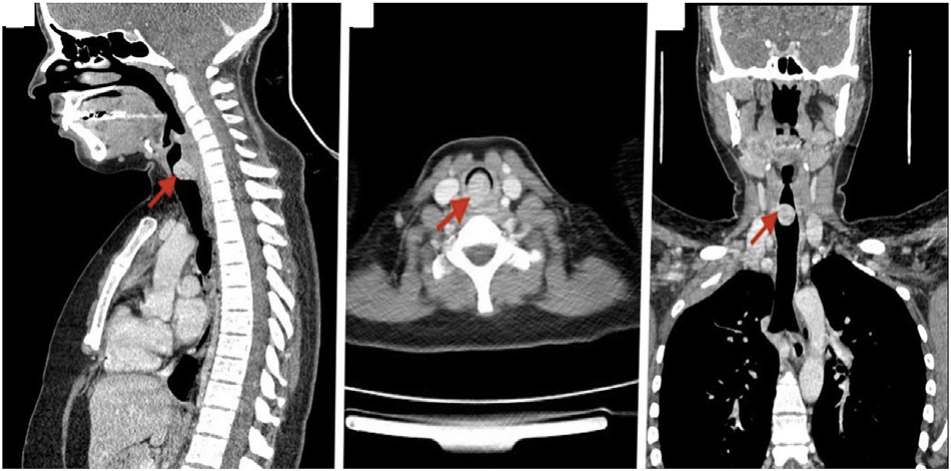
Thoracic CT scan with sections showing endotracheal tumor.
